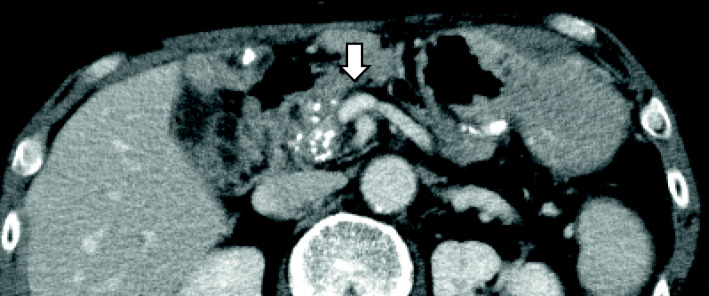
Findings of enhanced CT 3 years after surgery. The stenosis of the portal vein was improved
